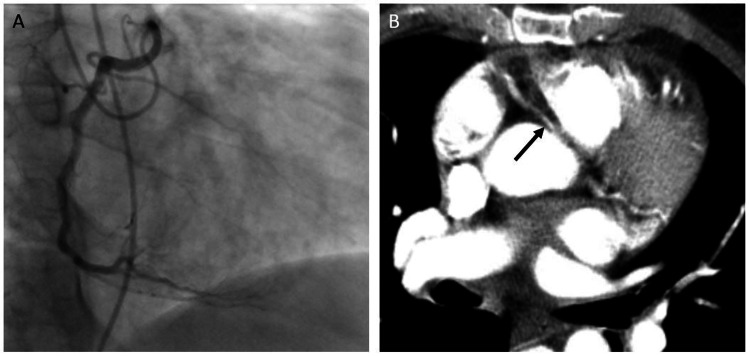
Imaging findings Coronary angiography (A) showing an anomalous RCA off the left coronary cusp with high anterior takeoff. Axial contrasted CT scan (B) of the chest showing an anomalous RCA arising from the left sinus of Valsalva coursing between the aorta and pulmonary artery with the proximal RCA with an oval/slit-like appearance suggesting an intramural course
